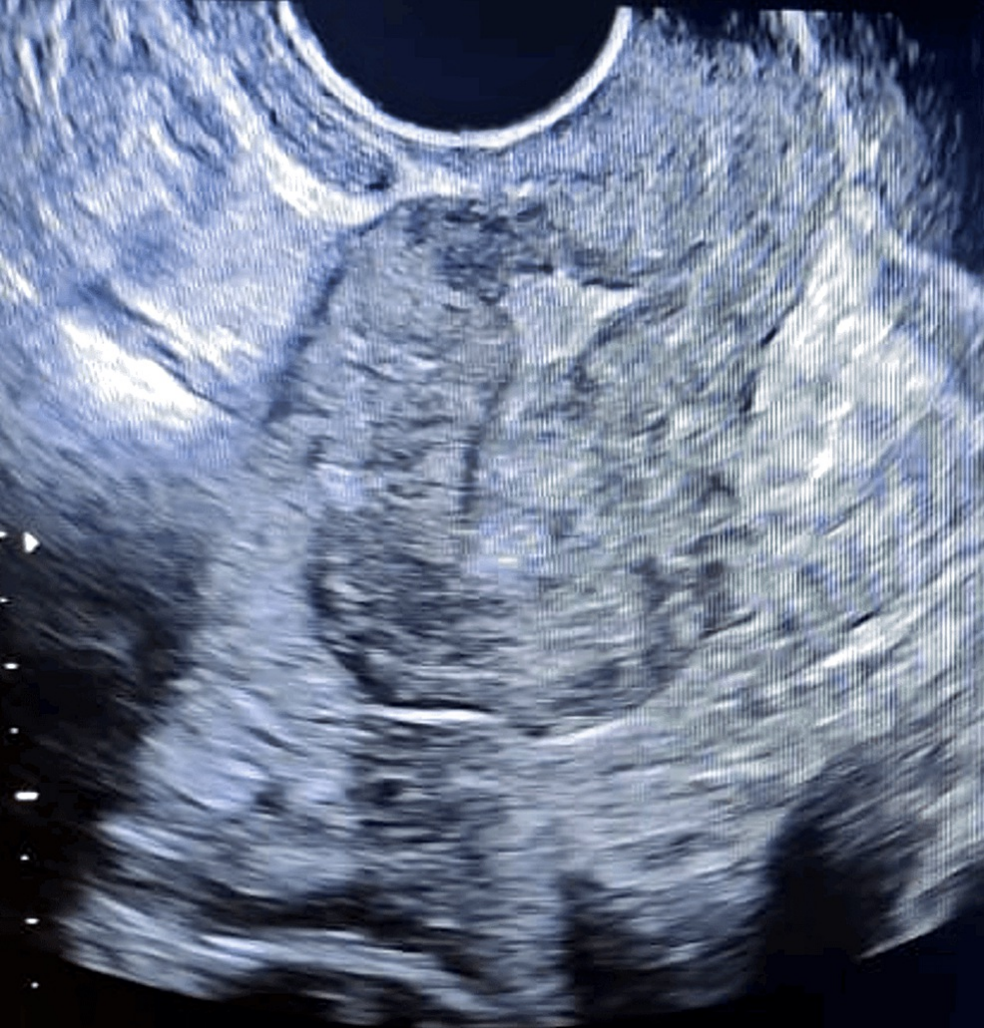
Showing ultrasonography preoperative Isthmocele
